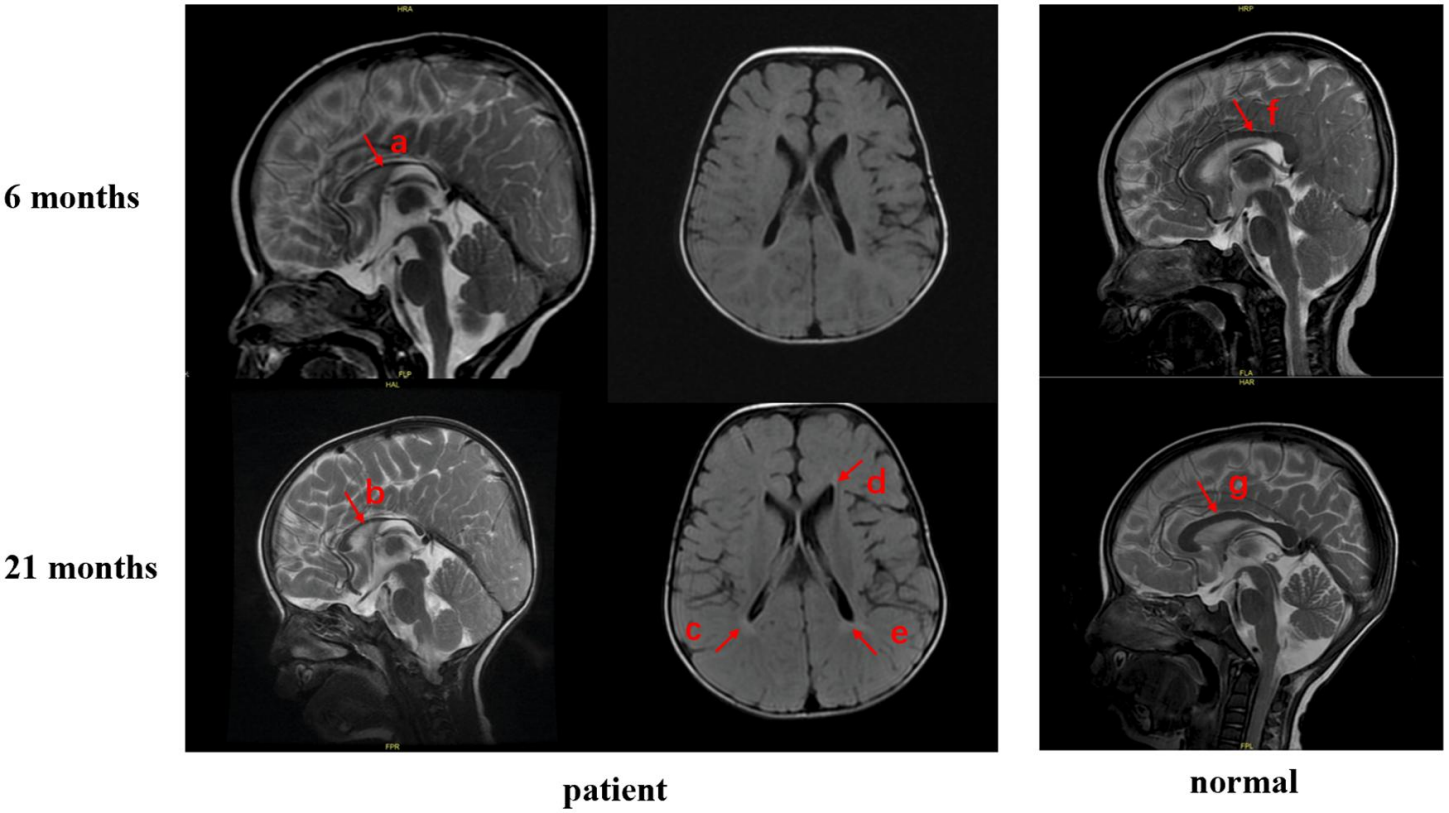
Brain MRI. (a) Corpus callosum of the patient at 6 months of age. (b) Corpus callosum of the patient at 21 months of age. (c–e) Abnormal signal intensity in the periventricular region of the patient at 21 months of age. (f-g) Corpus callosum of a healthy age-matched control.
